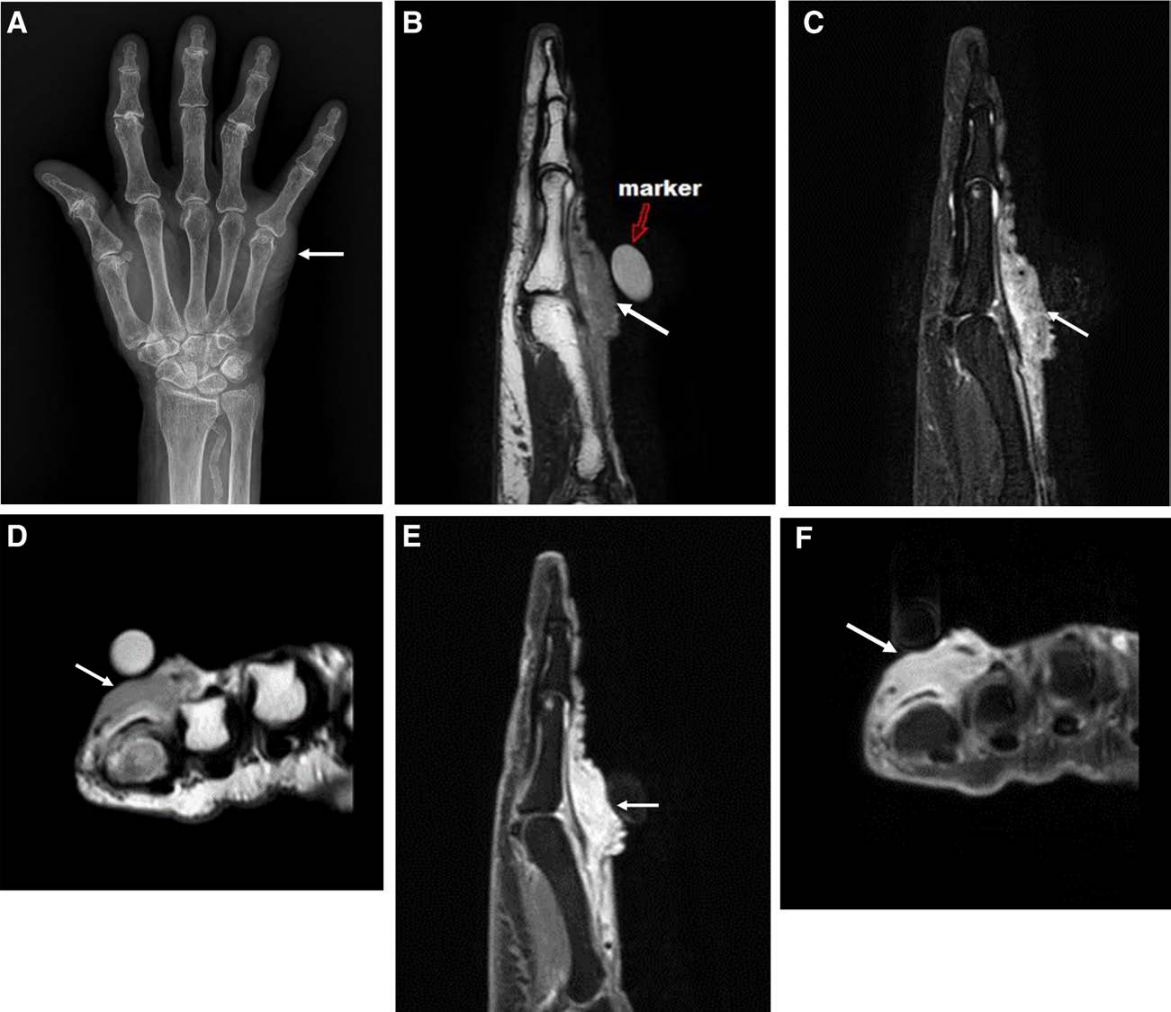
Plain anteroposterior radiograph of the hand shows focal soft tissue swelling (arrow) around metacarpal joint (MPJ) of the little finger (A). MRI of the hand demonstrates an ill defined soft tissue mass (arrows) with low T1 SI (B and D) and intermediate T2 SI (C) in the dorsal subcutaneous aspect of MPJ level in the little finger. Postcontrast fat suppressed T1WI (E and F) shows well, heterogeneously enhancement of the mass (arrows) with neurovascular infiltrative appearance. MRI = magnetic resonance imaging.
